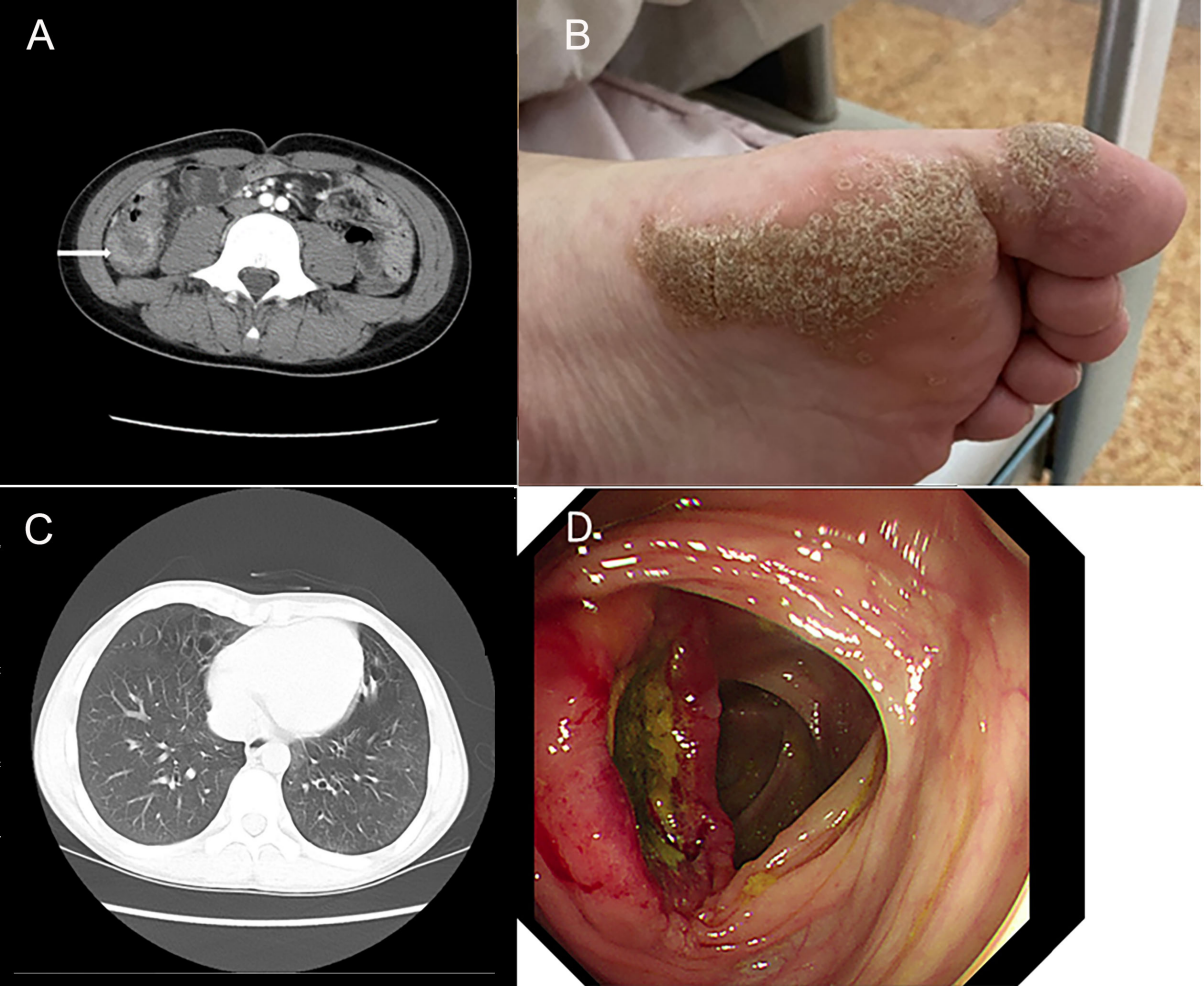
Clinical images show the clinical manifestation of the patient. (A) Computerized abdomen tomography (CT) revealed the thickened intestinal wall of ileocecum and patchy blurred shadows around it. (B) Skin lesions on the soles of the patient’s left foot. (C) Chest CT showed bronchiectasis in both lungs. (D) A huge ulcer with yellow pus moss on it of ascending colon on colonoscopy.
